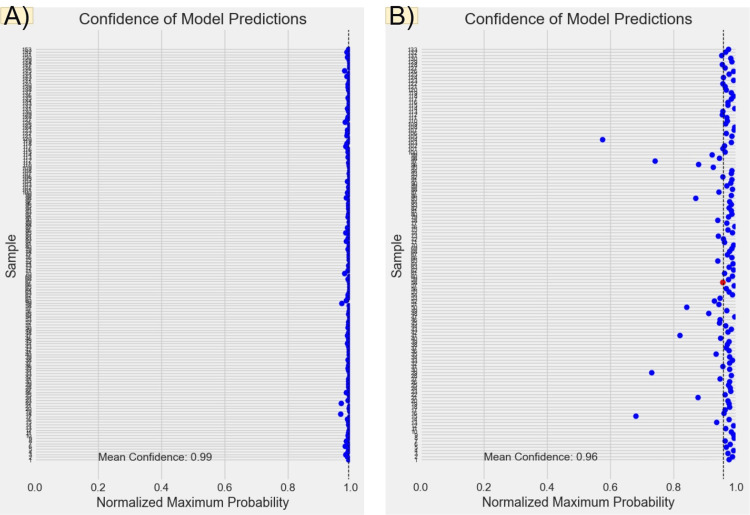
Predicted probabilities and confidence levels for inraoral and radiographic models. Panel (A) presents the predicted probabilities for each test sample from the intraoral model. The x-axis represents the normalized maximum probability values, while the y-axis corresponds to the test samples. This panel reflects the main model's confidence in its predictions. Panel (B) shows the predicted probabilities for each test sample from the radiographic model.
